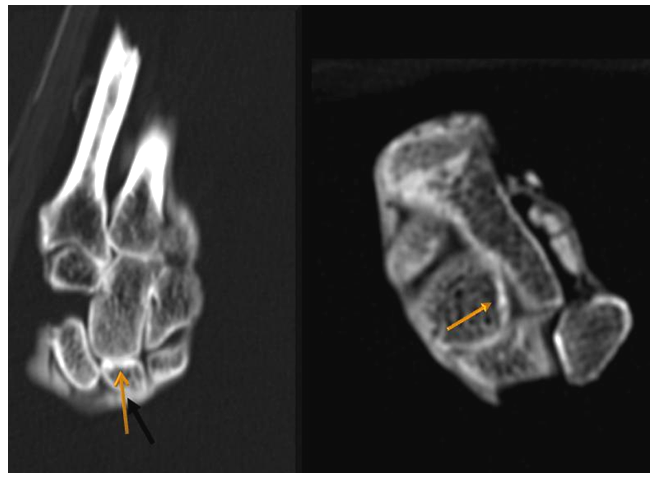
X-rays of Geheset's left hand. Minor sclerotic changes are indicated by the arrows.
